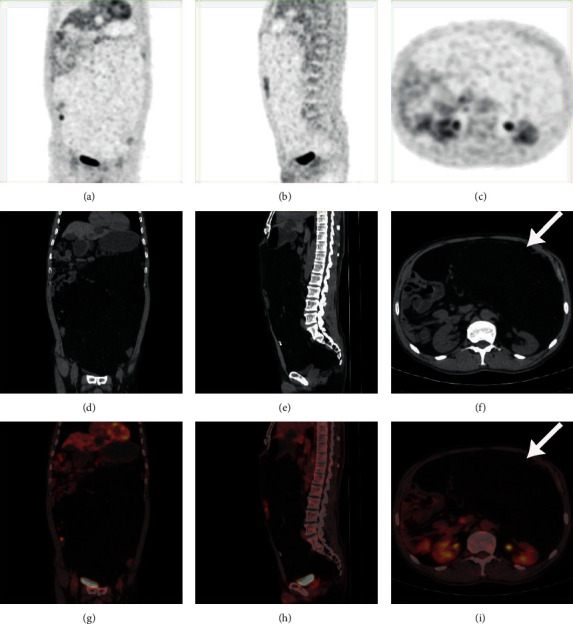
PET/CT images of a WDLPS patient with an SUVmax of 1.4 (white arrow).
